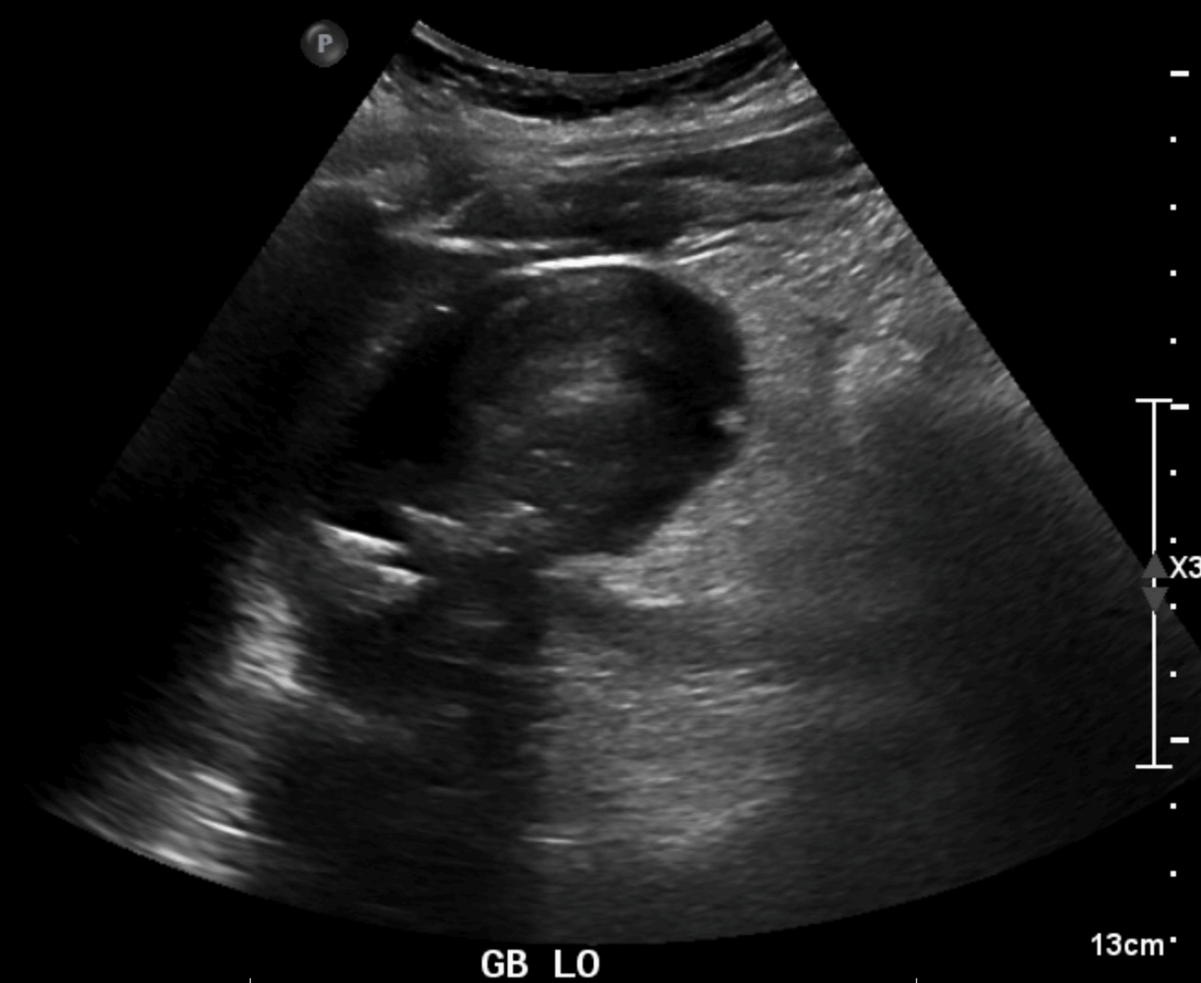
Right upper quadrant ultrasound image from radiologic ultrasound that was also ordered, showing gallstones and air shadowing from the GB wall, read via radiology as emphysematous cholecystitis.
